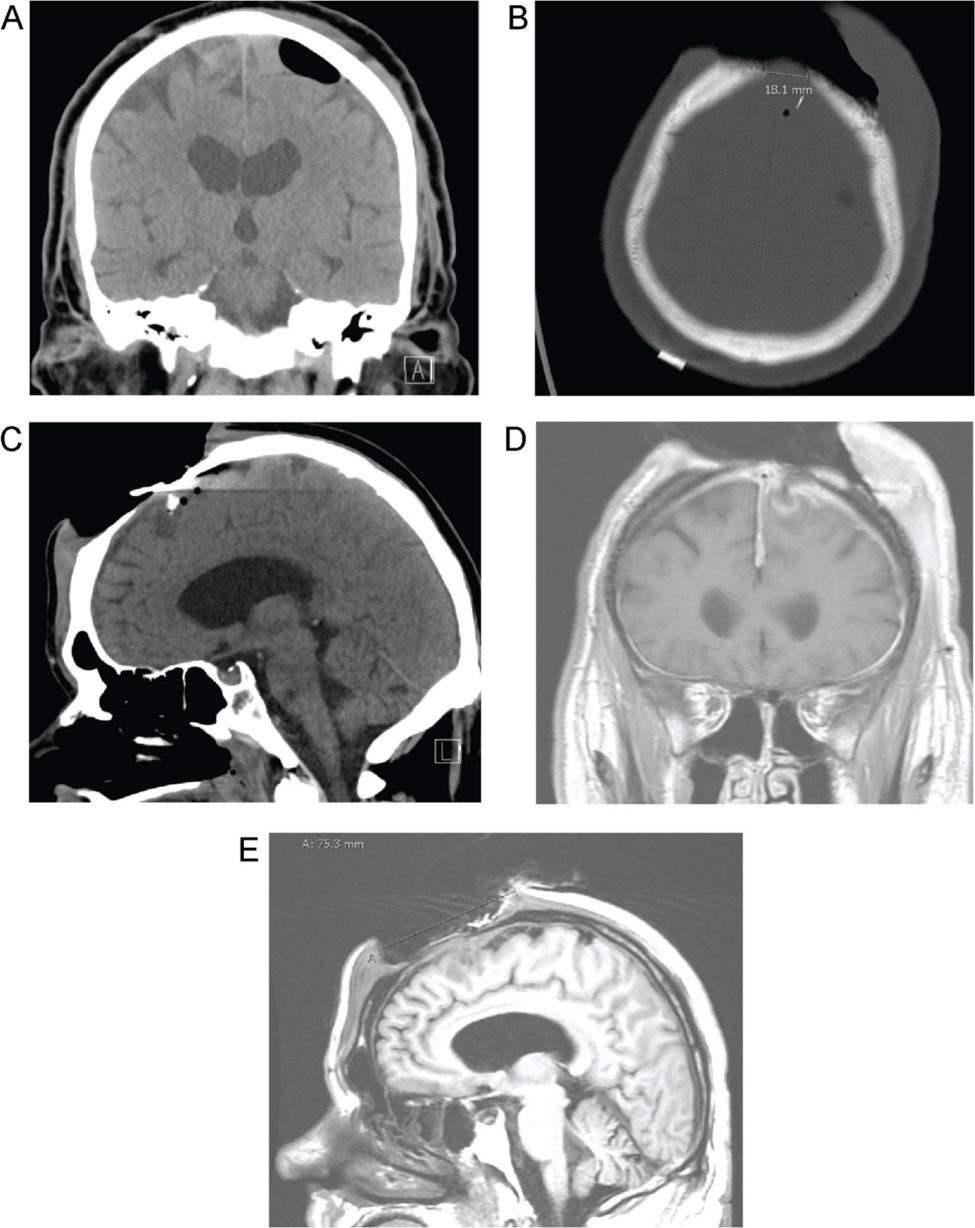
Imaging on initial presentation: A: Coronal CT demonstrating left parasagittal intracranial air as a sign of a dural defect; B: Axial CT (bone window) demonstrating the bone defect, a bone splinter and the depth of the skin defect; C: Sagittal CT again showing the bone splinter entering the frontal lobe as well as the sagittal extent of the scalp and bone defect; D: Coronal MRI showing gadolinium enhancement underneath the defect as a sign of encephalitis; E: Sagittal MRI demonstrating the extent of brain involvement
